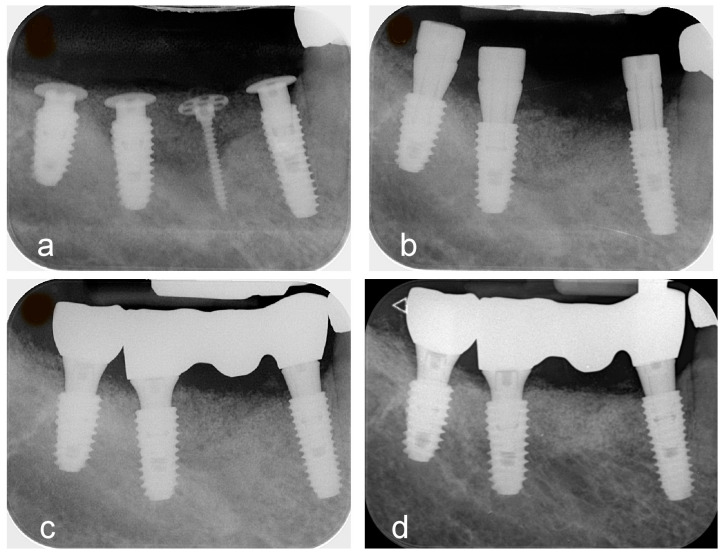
Serial radiographs. (a) Panoramic radiograph obtained immediately after surgery. (b) Radiograph taken 4 months postoperatively at the time of implant uncovering. (c) Baseline radiograph captured at the time of final prosthesis delivery. (d) Radiograph after 2 years of functional loading, demonstrating stable maintenance of the augmented ridge above the implant platform.
